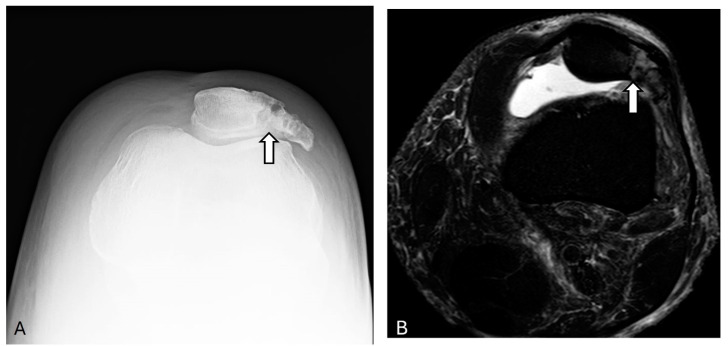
Multipartite patella. Knee X-ray (A) and axial proton density fat-suppressed image (B) reveal multipartite patella (arrows) with lateral irregularities and edema in the bone and lateral retinaculum suspicious for abnormal motion between the patellar and bipartite portion. The images were obtained from the University of Washington Medical Imaging database with the required permissions.
